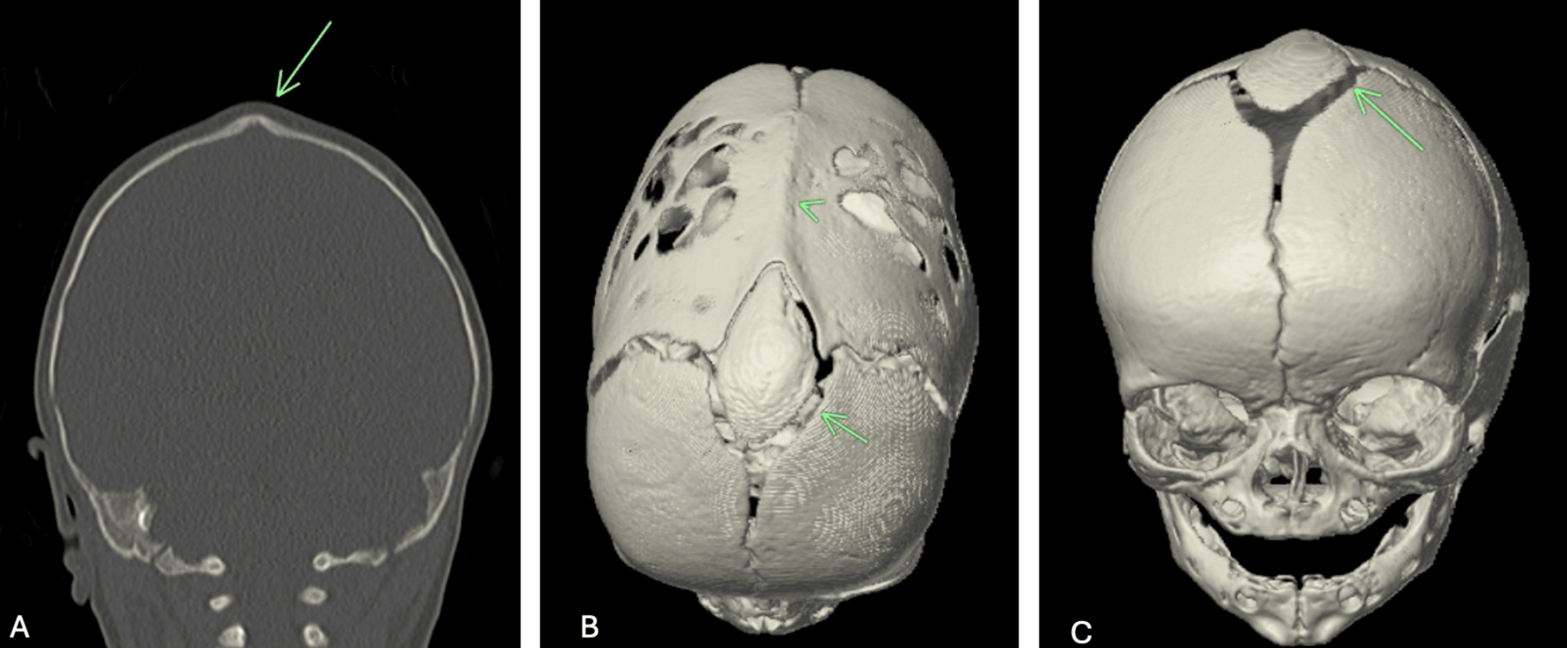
Three-dimensional computed tomography reconstruction of the skull demonstrating (A) complete fusion of the sagittal suture extending from the anterior to posterior aspect, presenting as an elevated osseous ridge indicative of sagittal craniosynostosis (arrow). (B) Axial view demonstrating a large, well-defined wormian bone measuring 4.3 × 2.5 cm occupying the anterior fontanelle region (arrow), with the fused sagittal suture visible posteriorly (arrowhead). (C) Coronal view highlighting the prominent anterior fontanelle wormian bone with intact cortical margins (arrow).
